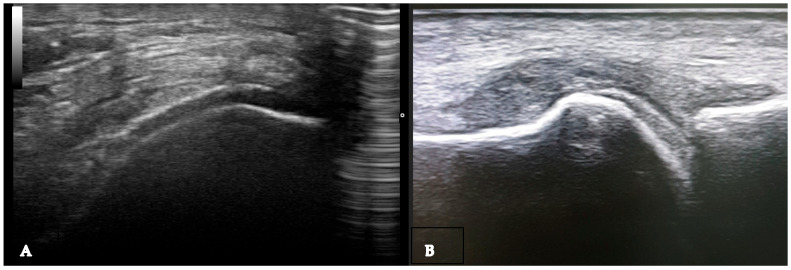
Ultrasound features of “double contour sign” visible on the cartilage surface of the femur (A) and first metatarsal bone (B).
